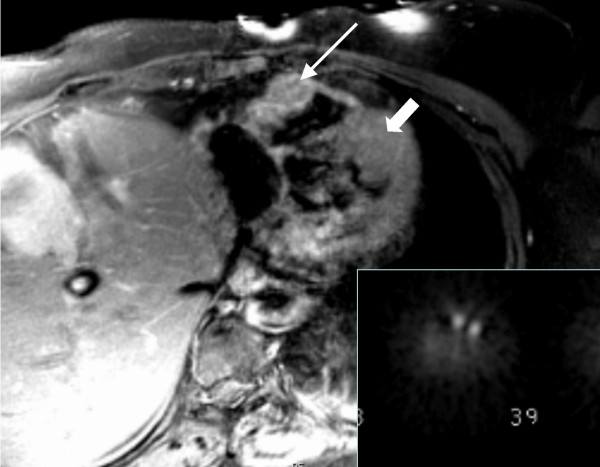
T1 gated axial MRI image demonstrating mass in the right ventricular wall (long arrow) and more poorly-defined lesion in the left ventricular wall (block arrow) corresponding to sites of abnormal radiotracer uptake on indium-111-pentetreotide scintigraphy (inset).
